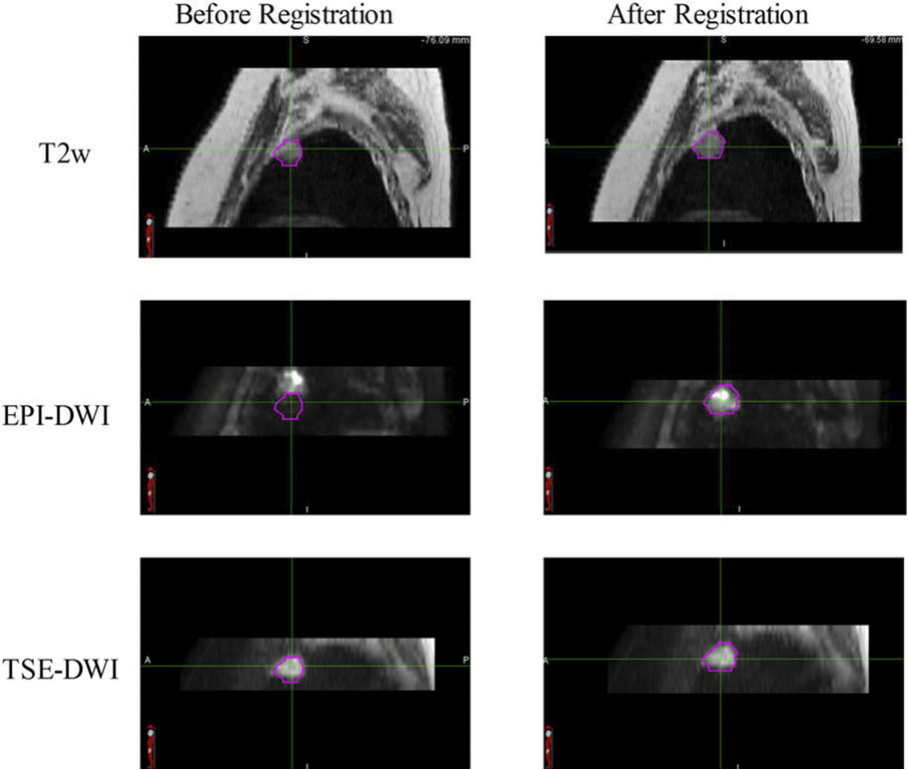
Sagittal orientation of a patient scan representing tumor location before and after manual registration. The figure shows T2w MRI (top row), EPI‐based DWI (middle row) and TSE‐based DWI (bottom row). EPI‐DWI shows a 1.5 cm shift in tumor center location because of susceptibility artifact shown by the location of the tumor contour on the EPI image. The tumor location is corrected after manual registration. The susceptibility artifact on TSE‐DWI is negligible.
